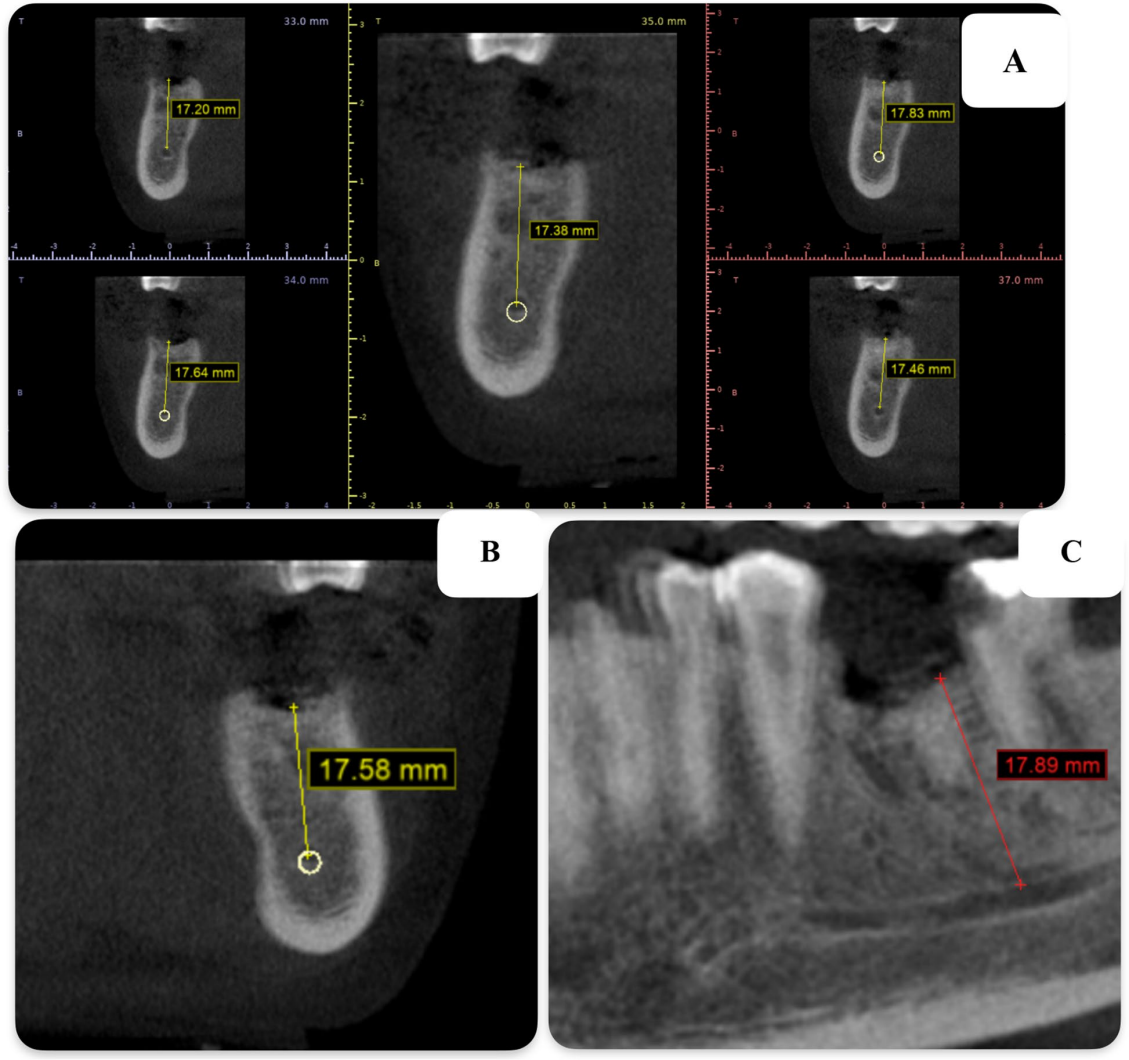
CBCT images of views utilized in measuring bone height A cross-section view B coronal view C panoramic view
